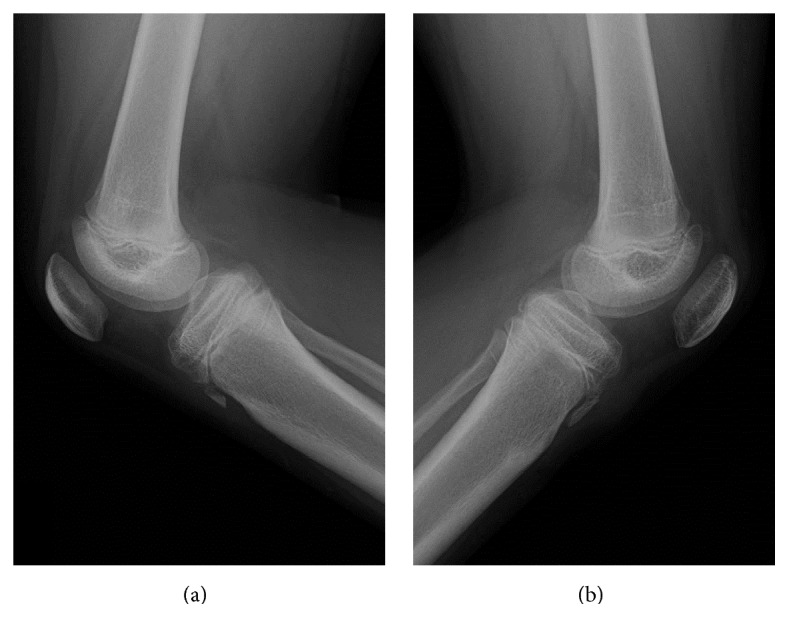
One year after surgery, lateral radiographs of the (a) right knee and (b) left knee.
